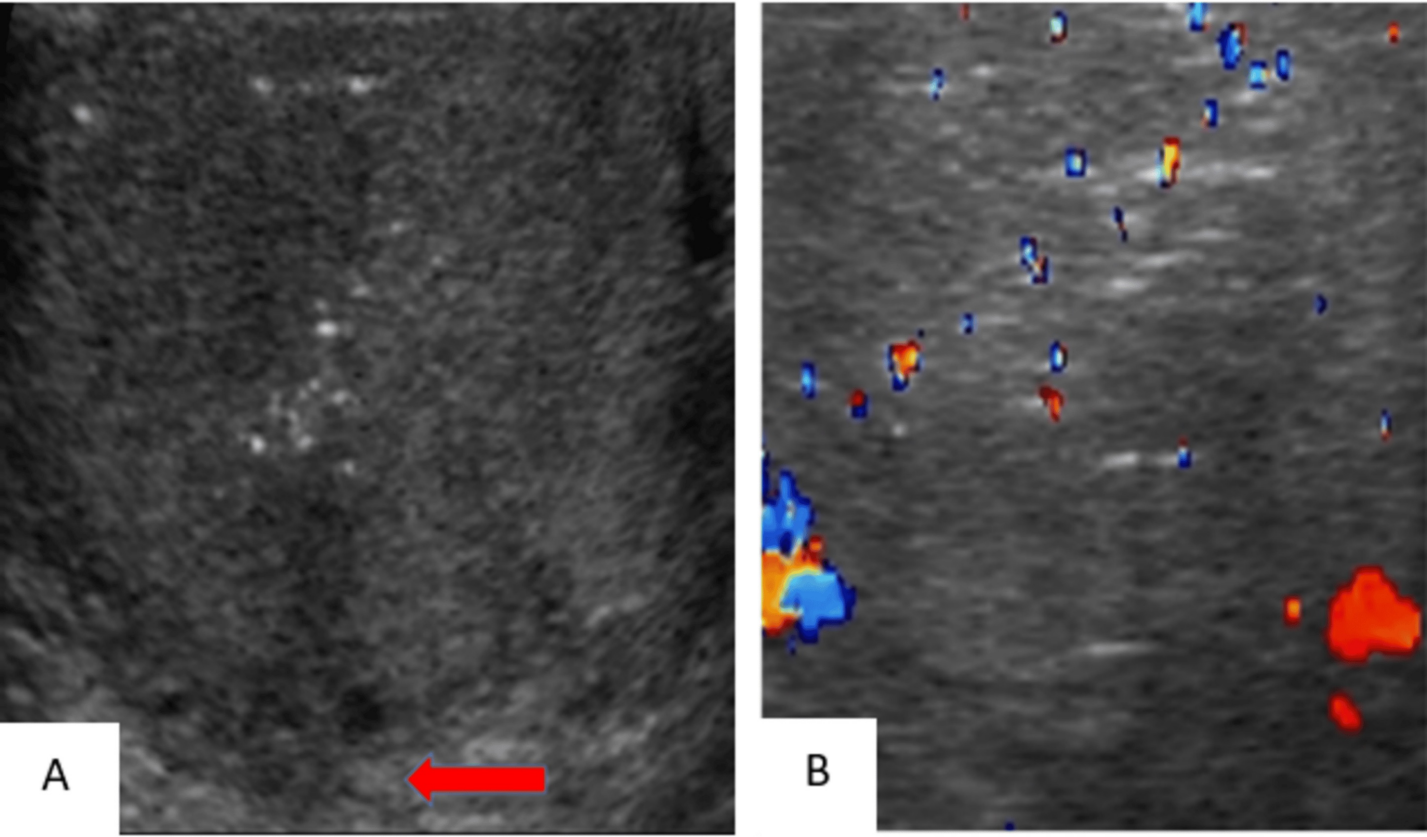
Testicular rupture with broken continuity of the tunica albuginea and irregularly outlined lower pole as seen on grayscale US (14A). Absent blood flow within a hematoma juxtaposed with preserved blood flow to the surroudning testicular tissue shown on color Doppler US (14B).
